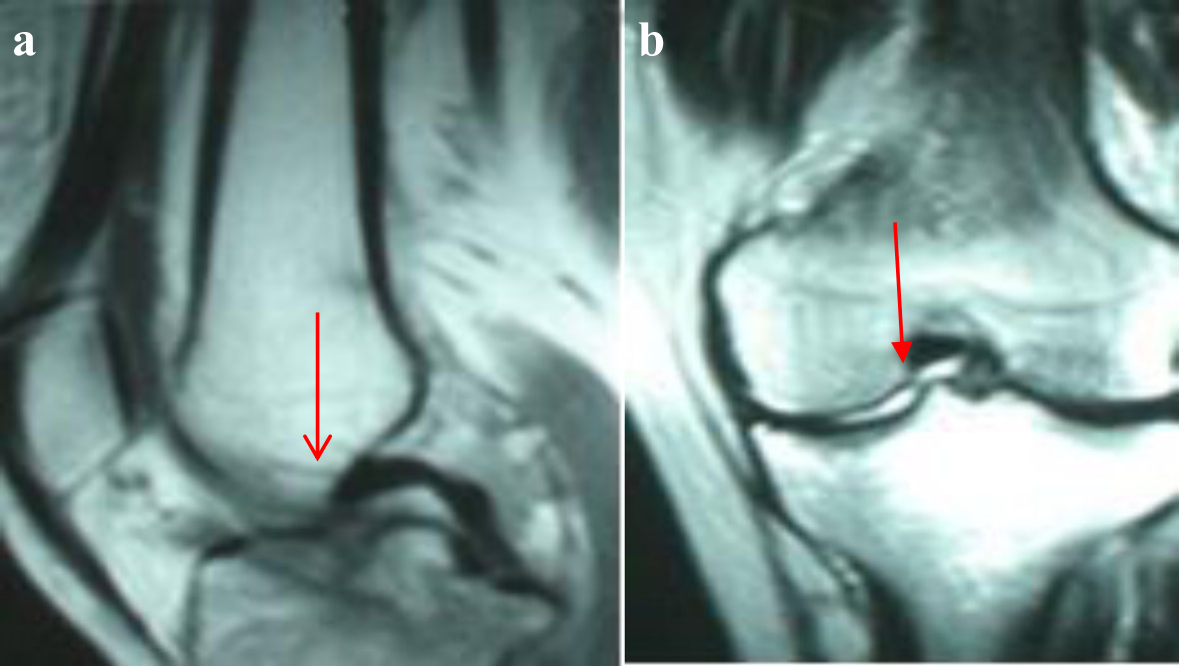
The Osteochondral fragment and its location on medial tibial plateau in magnetic resonance images. (a) *Red arrow* revealed the osteochondral fragment and its location on medial tibial plateau in sagittal magnetic resonance images. (b) *Red arrow* revealed the osteochondral fragment and its location on medial tibial plateau in coronal magnetic resonance images
